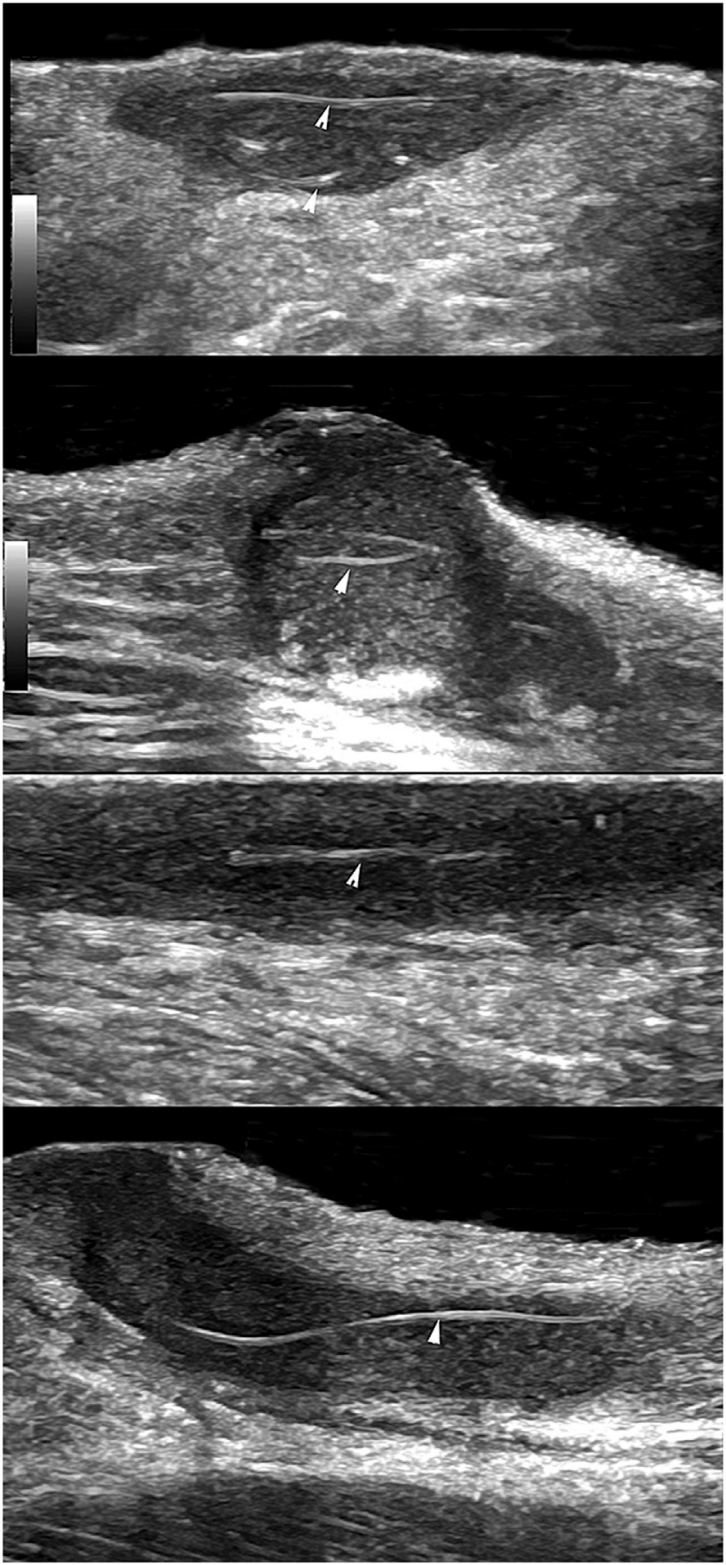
Using ultrasound to detect retained hair tracts (indicated by the arrows) within fistulae in hidradenitis suppurativa. This is indicative of regions that could flare with further inflammatory reactions and worsen extent of the disease. The mean length of hair tracts was 4.4 mm with a wide dispersion (SD of 6.9 mm) and a range of 2–31.7 mm. Images were taken with a General Electric Logic E9 with a maximum ultrasound bandwidth of 18 MHz. Permissions obtained to reprint from Wortsman and Wortsman (61).
